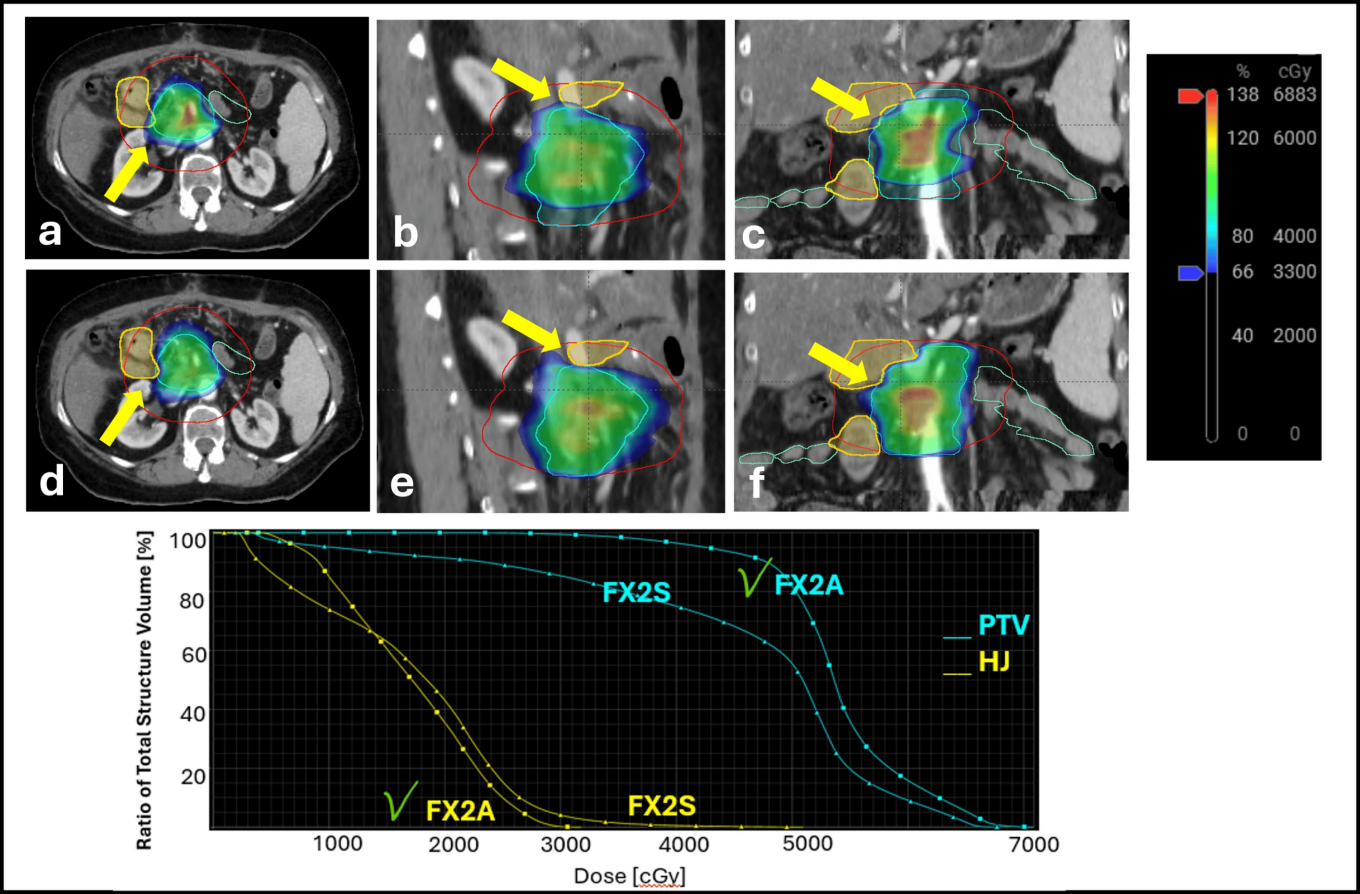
Axial, sagittal, and coronal views of daily CBCT for FX2 of the scheduled plan (top row a-c) and adapted plan (middle row d-f), respectively, with color wash dose overlayed on the scans. Yellow arrows point to the area where the 33 Gy OAR dose tolerance is violated in the scheduled plan for the HJ (yellow contour). On the other hand, in the adapted plan, 33 Gy does not overlap with HJ. Red contour represents the 3 cm contour ring, magenta represents the liver, and orange and green contours represent large and small bowels, respectively. Bottom row is the DVH plot that shows the max dose constraint to HJ was violated in the scheduled plan(FX2S), while this constraint was met in the adapted plan (FX2A). PTV (cyan) is shown in triangle-line for the scheduled plan and square-line for the adapted plan. The green check mark sign in the DVH plot next to FX2A indicates that these constraints were met in the adaptive plan. CBCT: cone beam computed tomography, OAR: organ-at-risk, HJ: hepaticojejunostomy, DVH: dose-volume histogram, PTV: planning target volume
